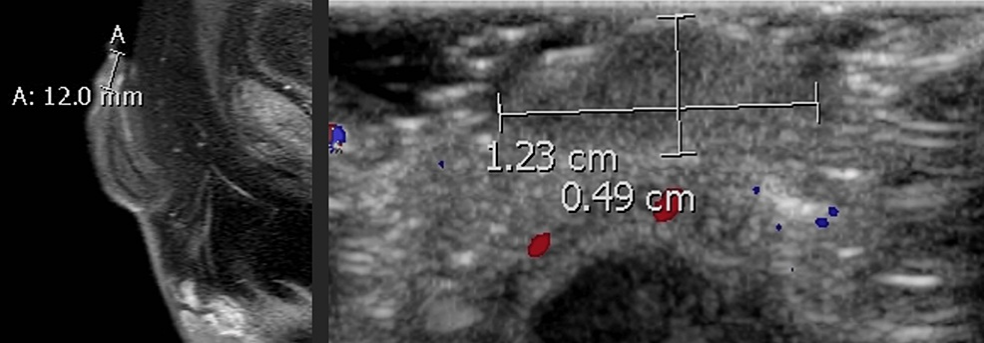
MRI and ultrasonography examinations of the lesion.
